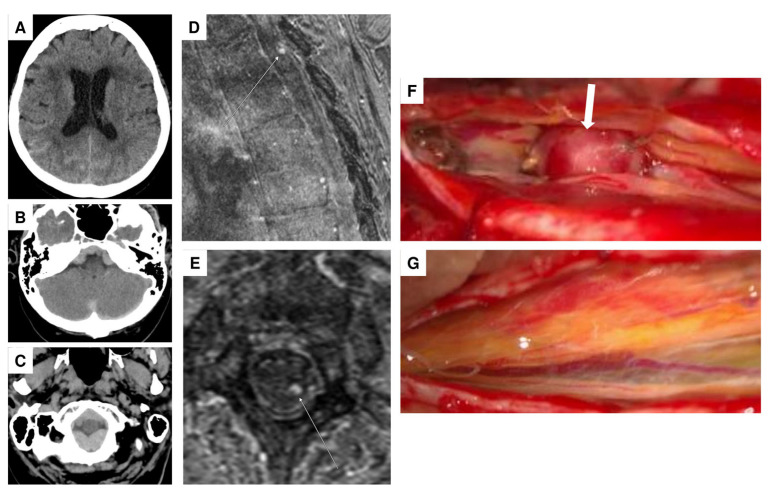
Noncontrast head CT in the transversal plane showing minor subarachnoid hemorrhage, primarily in the right temporoparietal region (A), and distinct subdural hemorrhage in the posterior fossa, particularly involving the craniocervical junction (B,C). High-resolution contrast-enhanced MR angiography (ceMRA extreme) in the sagittal (D) and transversal (E) planes, showing a suspected perimedullary aneurysm dorsolaterally on the left at the T2 level (arrows), measuring approximately 3 mm in diameter. Intraoperative visualization of the aneurysm ((F), arrow). Post-resection view following coagulation of the feeding artery, showing no residual aneurysm (G).
